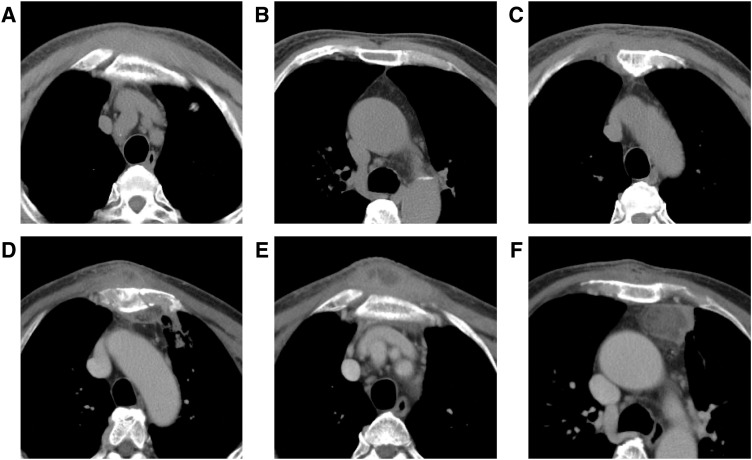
The first CT scan, which was performed at a local clinic, showed no specific findings in the subcutaneous tissue of the anterior chest (A), anterior mediastinum (B), or sternum (C). The 2nd CT scan, which was performed 13 days after the previous CT scan, showed destruction of the sternum (D) with the abscesses in the subcutaneous tissue of the anterior chest (E) and on the left side of the anterior mediastinum (F).
